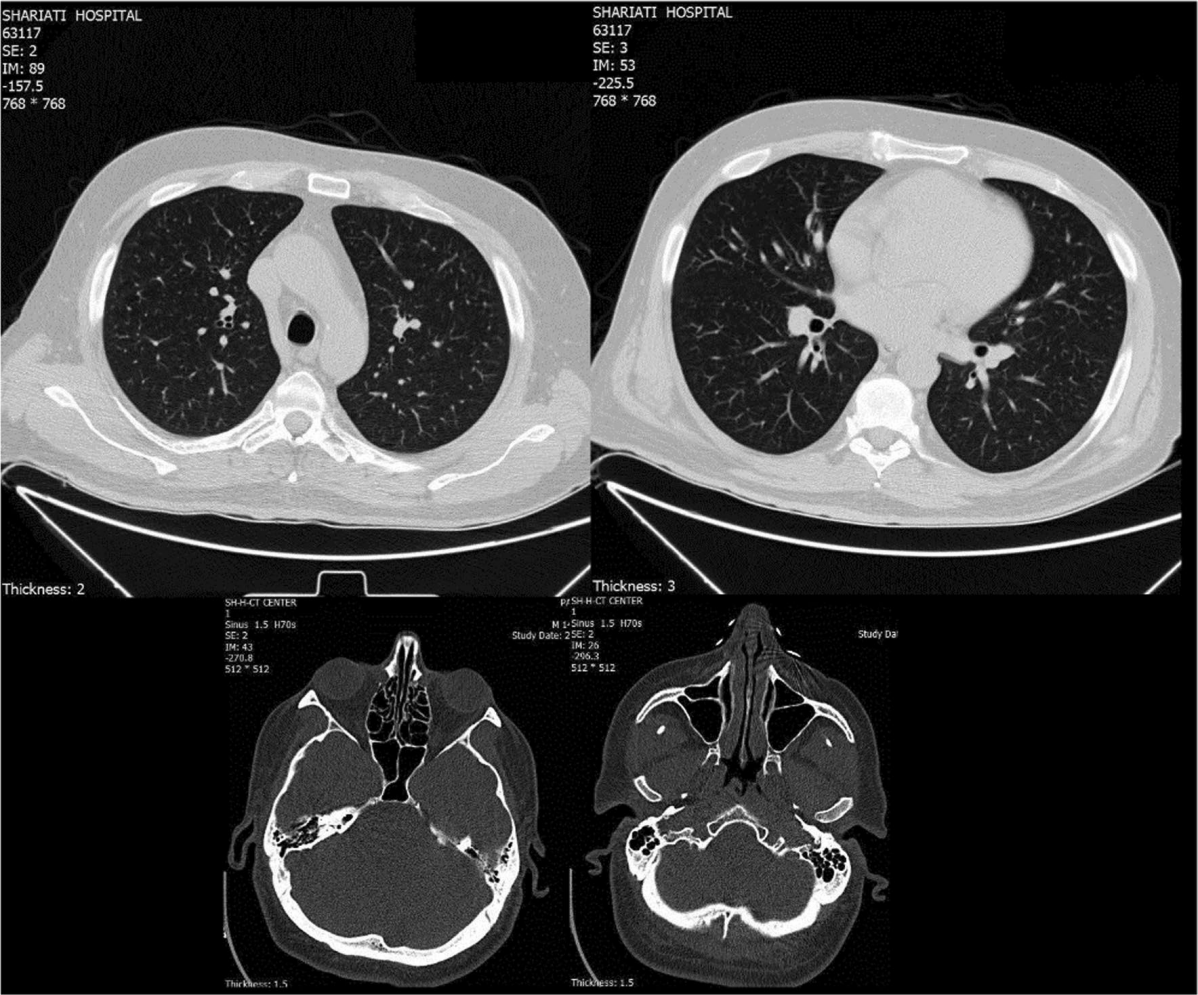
Normal computed tomography (CT) scan of lung and paranasal sinuses of 48-year-old male suspicious of vasculitis, with no evidence of alveolar hemorrhage, nodules, or cavitation
